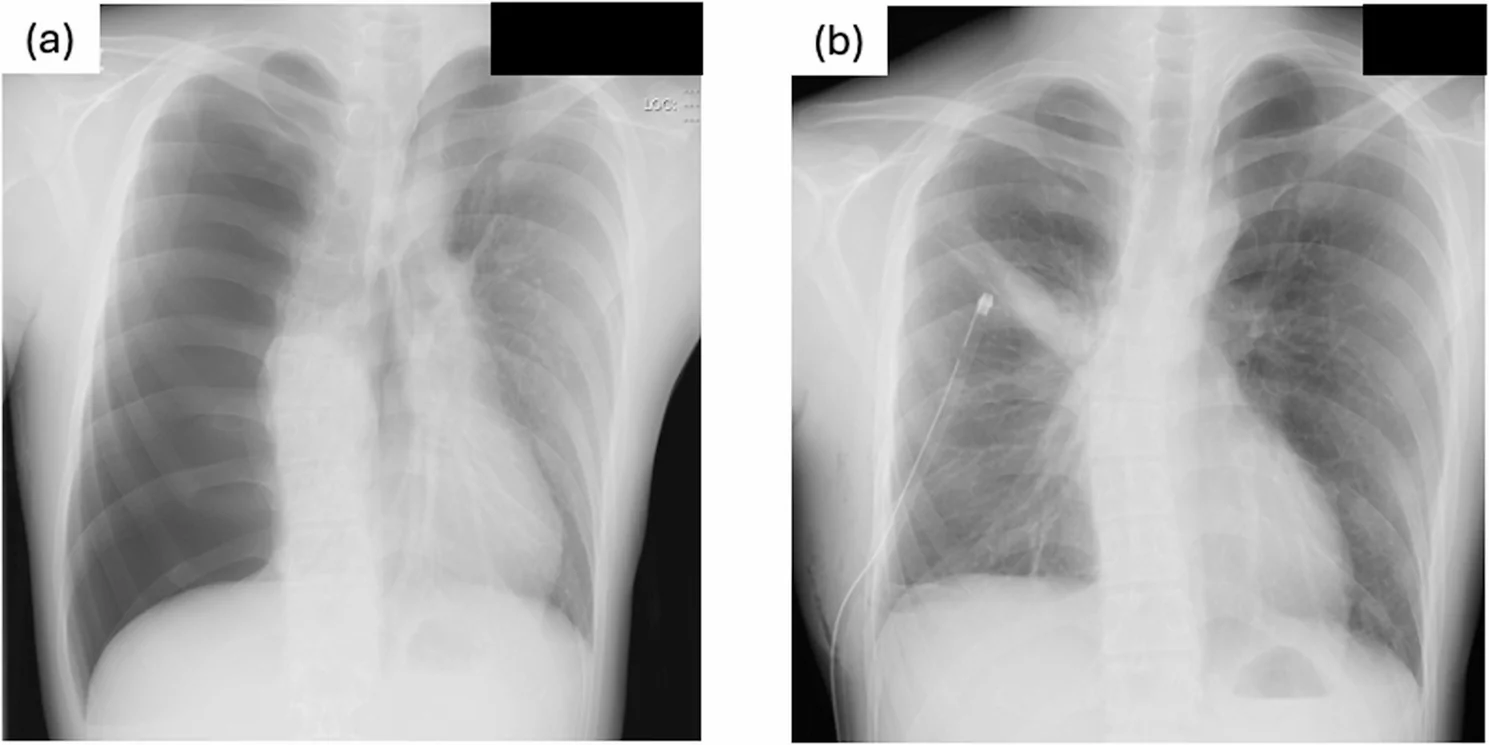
a Chest radiograph showing complete collapse of the right lung with mediastinal shift to the left. b On the day after chest tube drainage, lung re-expansion was obtained, but cord-like atelectasis was observed in the right middle lung field
